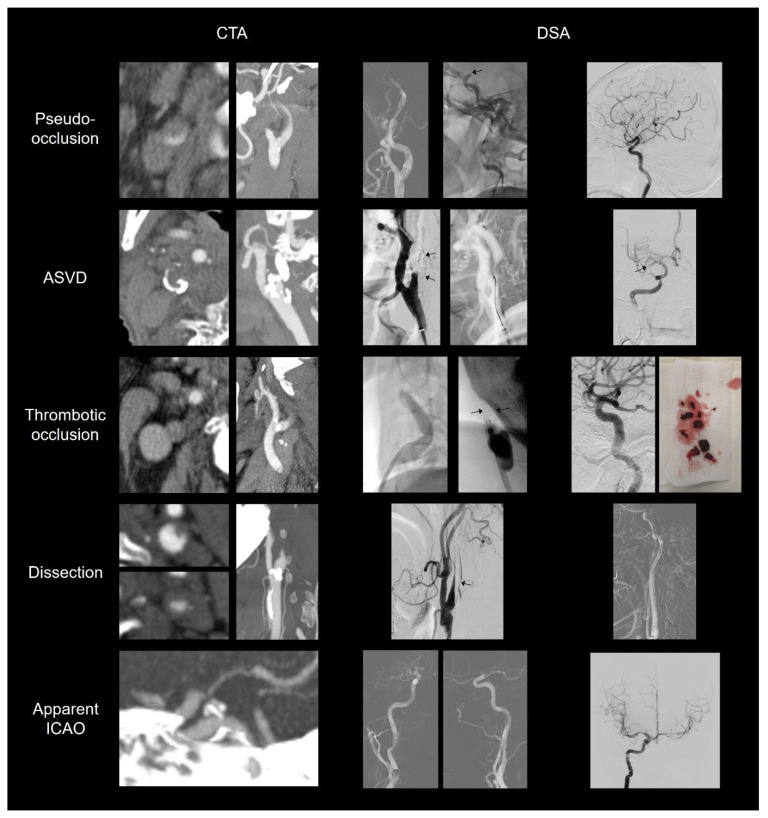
Representative computed tomography angiography (CTA) images and digital subtraction angiography (DSA) findings of internal carotid artery occlusion (ICAO) etiologies. First row: A patient with ICA terminus occlusion shows non-contrast filling of the left cervical ICA with a flame shape. In the axial image, the contrast is in the dependent portion, with an ill-defined margin. On DSA, delayed angiogram confirms the occlusion site at the ophthalmic ICA (arrow). After recanalization, the ICA shows no underlying stenosis. Second row: CTA shows ICAO due to a low-density plaque with dense calcification. The DSA image shows a blunt-shaped ICA occlusion. The wire had difficulty passing through the occluded segment. After carotid stenting, the aspiration catheter could pass through the stent and navigate to the intracranial tandem occlusion present in the middle cerebral artery (arrow). Third row: Images of a patient with ICA terminus occlusion with a blunt-shaped proximal ICA. On DSA, the occlusion started from the mid-cervical ICA. Upon contact aspiration using a large-bore aspiration catheter, a thrombus was retrieved while attached to the catheter tip (arrows). Multiple uses of contact aspiration resulted in the successful removal of a large amount of thrombus filling from the mid-cervical ICA to the ICA terminus. Fourth row: Images of a patient with ICA terminus occlusion. The CTA image shows a beak shape and circumscribed margin of cervical ICA. The common carotid angiogram shows a flame-shaped appearance with an intimal flap. The false lumen with stagnated contrast media is located posteriorly (arrow). After placing the guiding catheter in the true lumen, the roadmap image shows a dissecting flap and intracranial tandem occlusion. Bottom row: Images of a patient with apparent ICAO. CTA and DSA show ICA occlusion distal to the posterior communicating artery orifice. The contrast reaches the intracranial ICA and fills the ophthalmic and posterior communicating arteries.
